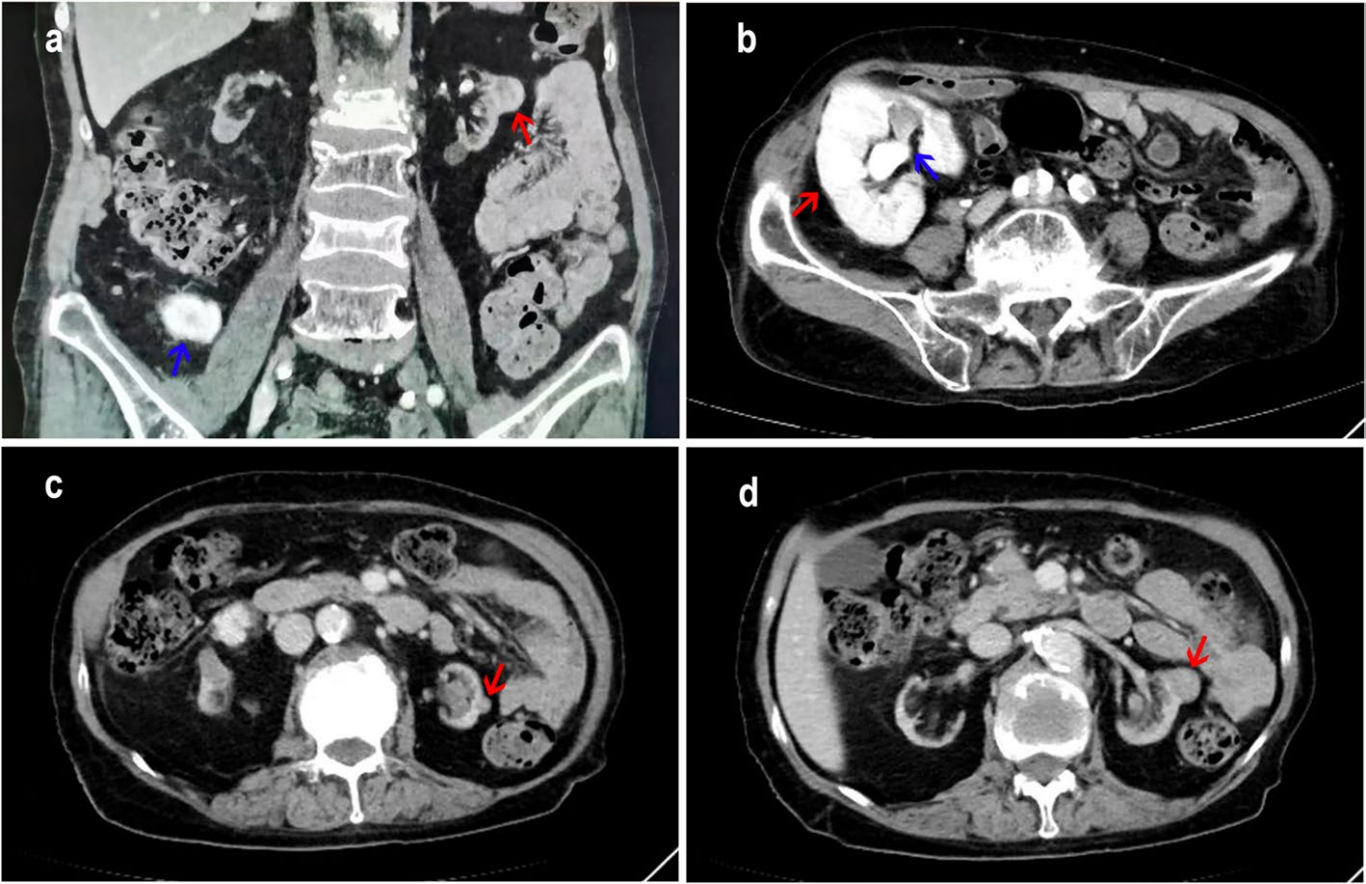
Enhanced CT findings in the patient. a The transplanted kidney shadow in the right iliac fossa (blue arrow). A slightly low-density shadow in the autologous left kidney, with some protrusion beyond the kidney contour (red arrow). b The transplanted kidney in the right iliac fossa (red arrow), with dilatation of the renal pelvis and some renal calyces which also contained an effusion (blue arrow). c Small rounded, slightly low-density shadows (mass (I)) in the autologous left kidney (red arrow). d Large rounded, slightly low-density shadows (mass (II)) in the autologous left kidney (red arrow)
